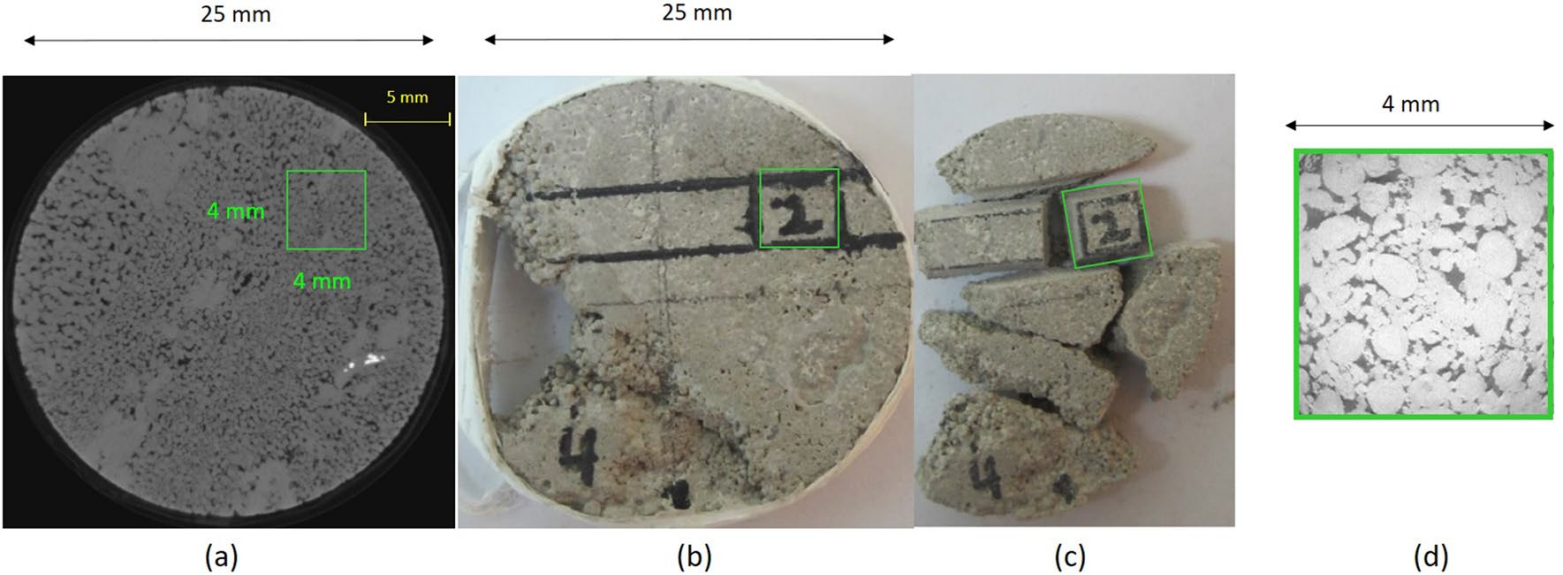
Subset selection and image acquisition at high resolution for Sample S_1_: (a) Location of 4 mm^3^ extracted subset on 3D X-Ray MCT at 20 μm resolution, (b) subset location on real core plug, (c) Subset extraction, and (d) Subset scanned at 4 μm resolution.
